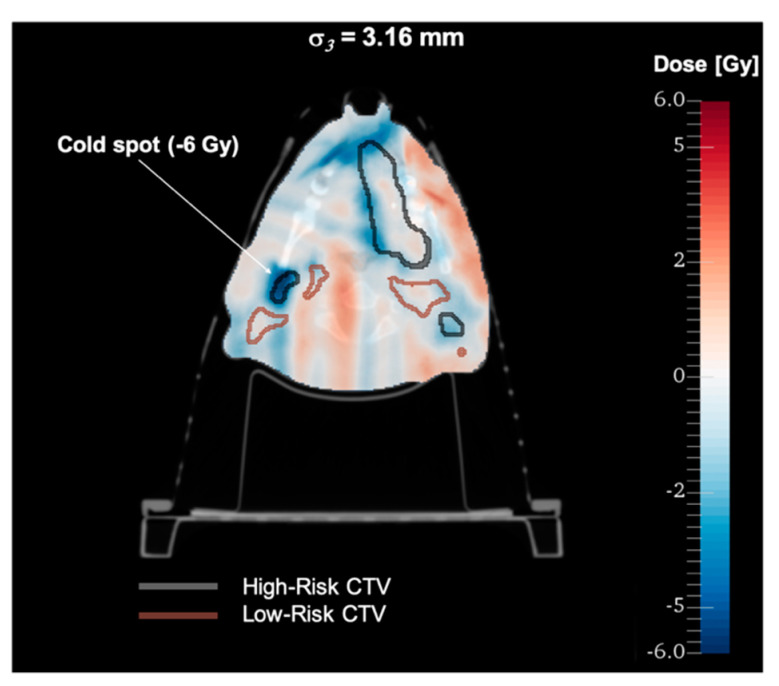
Difference in accumulated dose distribution for the largest CT-on-rails offset scenario (*σ*_3_ = 3.16 mm) with respect to CBCT-based online adaptation with no offset.
